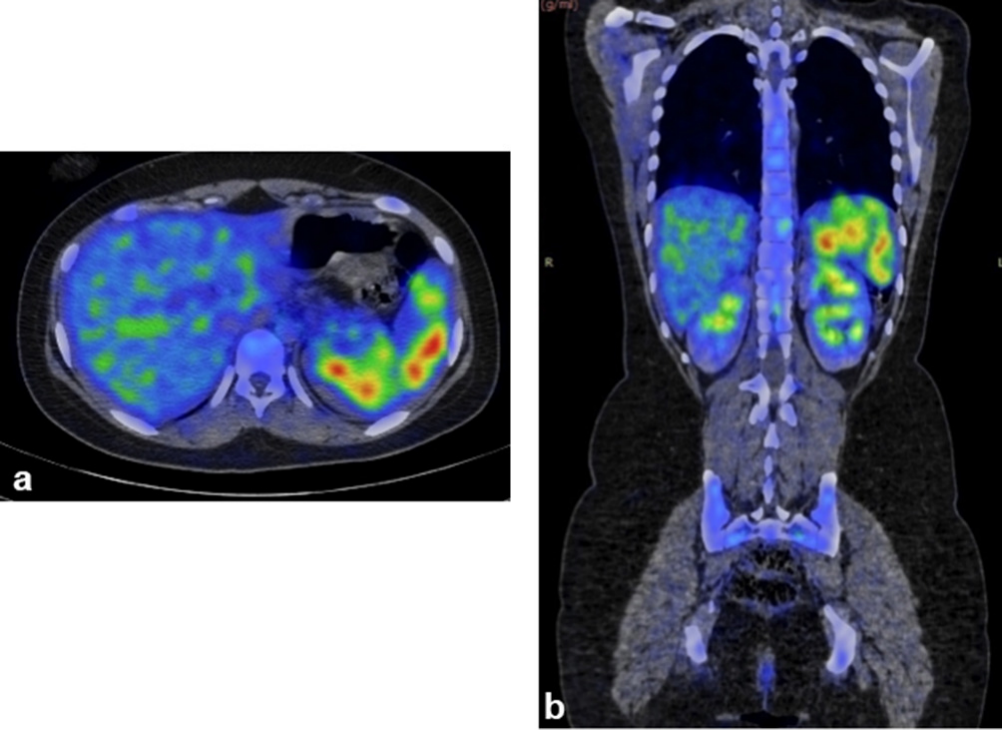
Fused fluorodeoxyglucose-positron emission tomography-CT (axial and coronal) images showing multiple focal fluorodeoxyglucose-avid nodular lesions in spleen.
